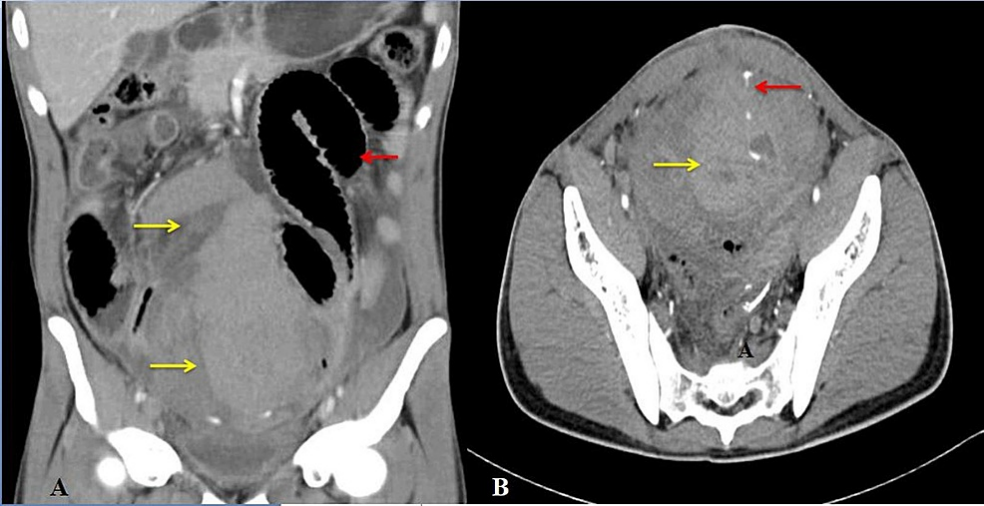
Contrast-enhanced computed tomography of the abdomen. (A) (Coronal view) Heterogeneously enhancing hematoma in abdomen and pelvis (yellow arrow) displacing bowel loops (red arrow). (B) (axial view) Heterogeneously enhancing hematoma in abdomen and pelvis (yellow arrow) with active contrast extravasation from the branch of the inferior epigastric artery from the abdominal wall (red arrow).
